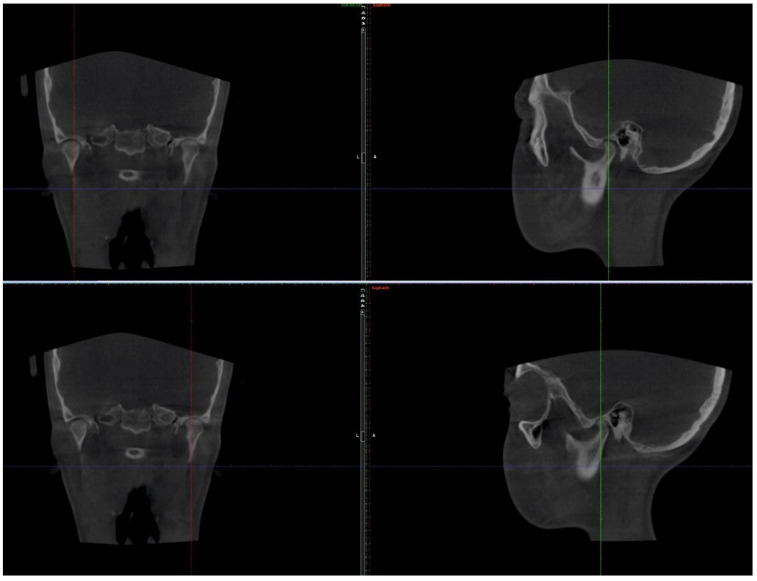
TMJ aspect on CBCT at the one-year follow-up appointment.
